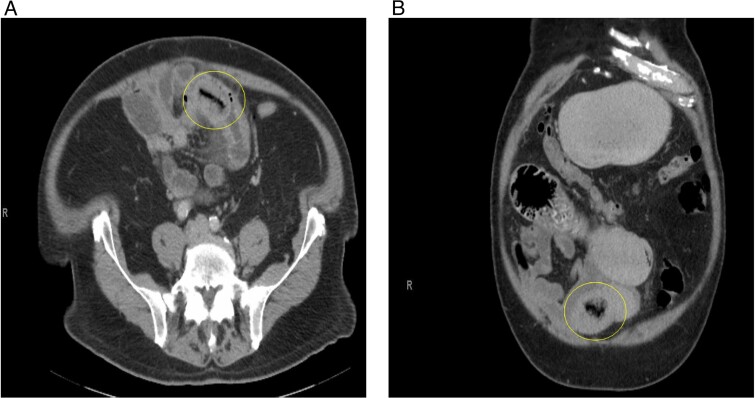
(A) Initial CT abdomen (transverse view) revealing what was thought to be intussusception but turns out to be primary enterolith with an air filled core. (B) Initial CT abdomen (coronal view) revealing what was thought to be intussusception but turns out to be primary enterolith with an air filled core.
